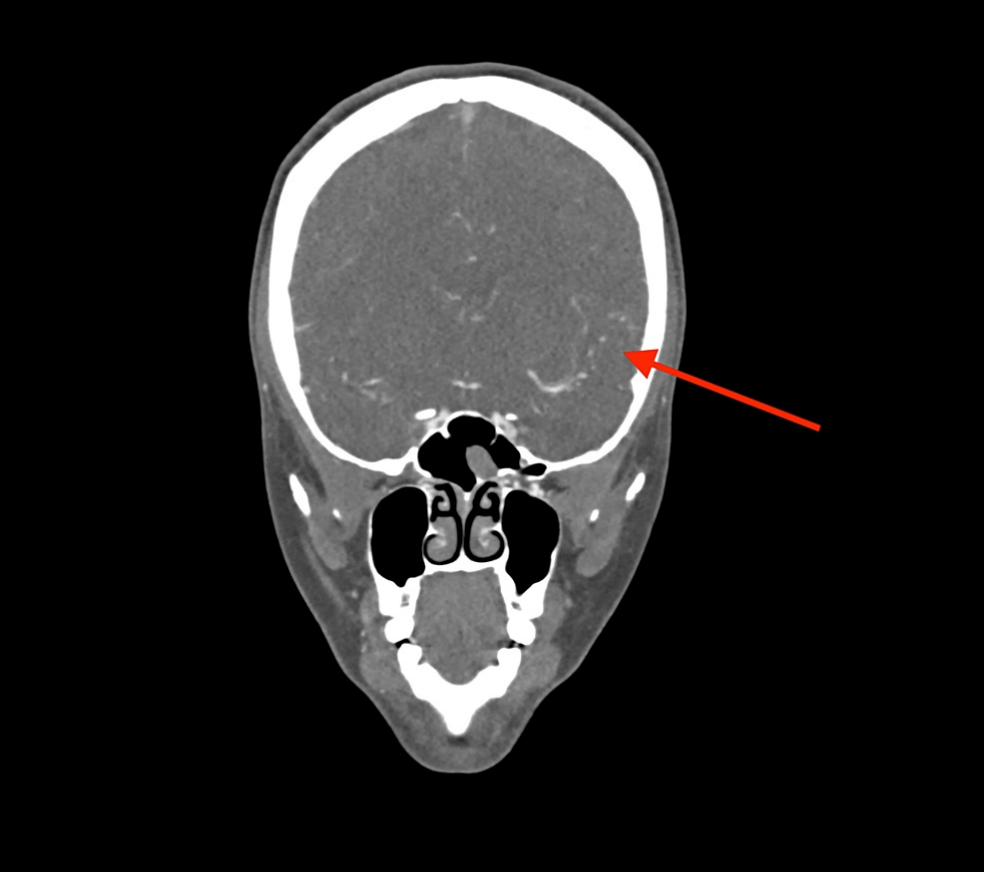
CT angiogram of the head and neck showing mild contour irregularity along the distal M1 segment of the left middle cerebral artery, suggesting underlying vasospasm (arrow).
